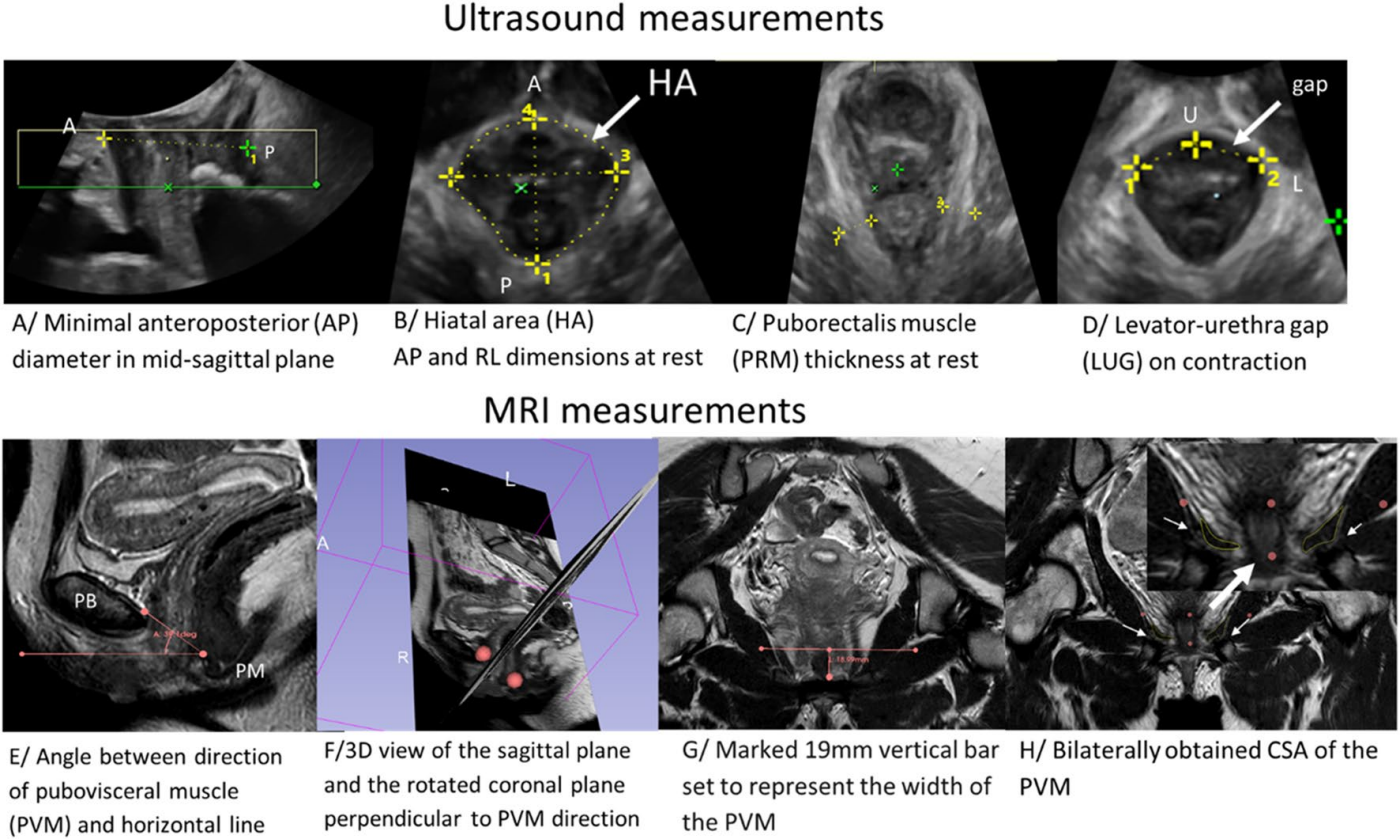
The representative figures of ultrasound (US) and magnetic resonance imaging (MRI) measurements. Figure A–D represents measured ultrasound dimensions. Figure E–H represents individual steps of one MRI measurement. The Figure H shows visible difference in left and right sided pubovisceral muscle cross sectional area on MRI. In the right upper corner of the Figure H is larger magnification. Abbreviations: A – anterior, P – posterior, HA – hiatal area, U – urethra, L – levator ani muscle, PB – pubic bone, PM—perineal membrane, PRM – puborectalis muscle, PVM—pubovisceral muscle
